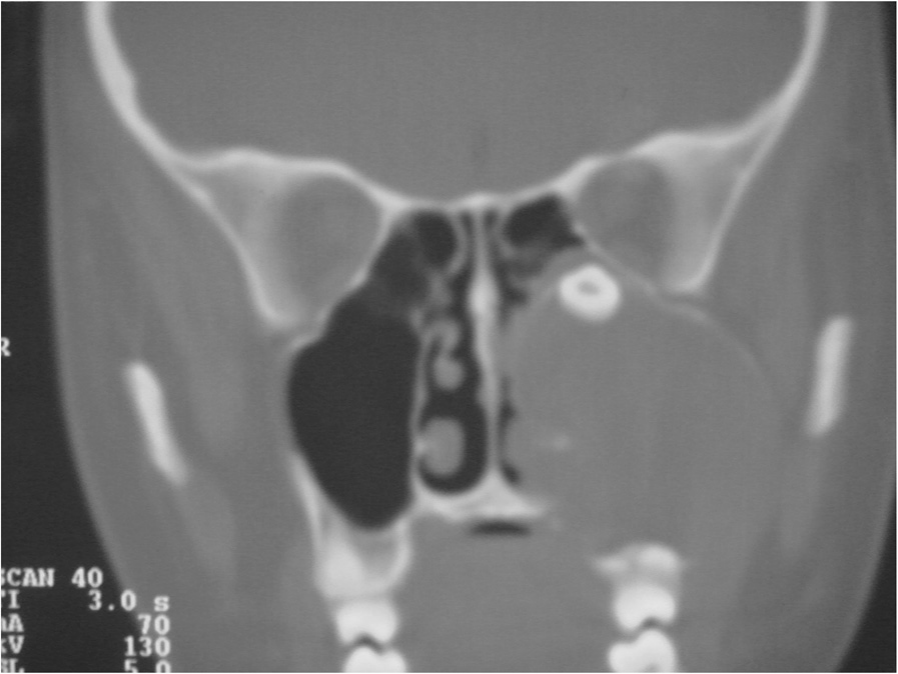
Computed tomography scan: coronal sections.
